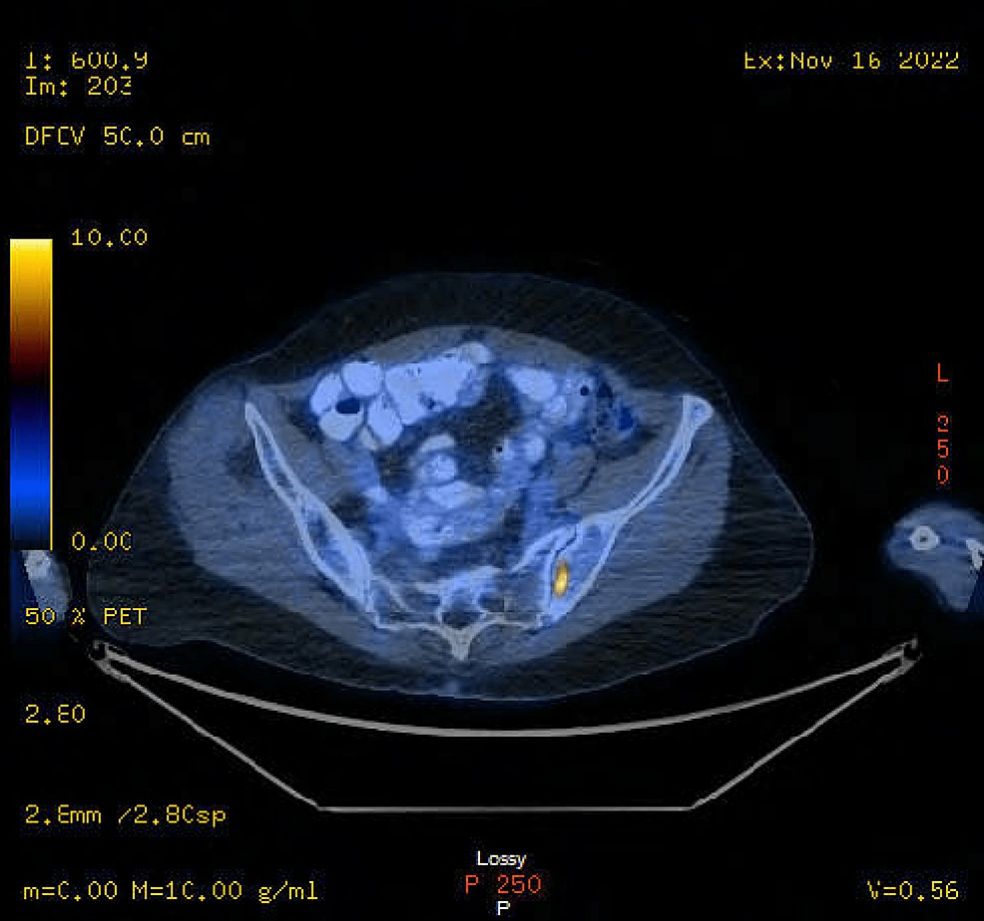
FDG-PET showing left femur involvement. FDG-PET: Fluorodeoxyglucose Positron Emission Tomography.
